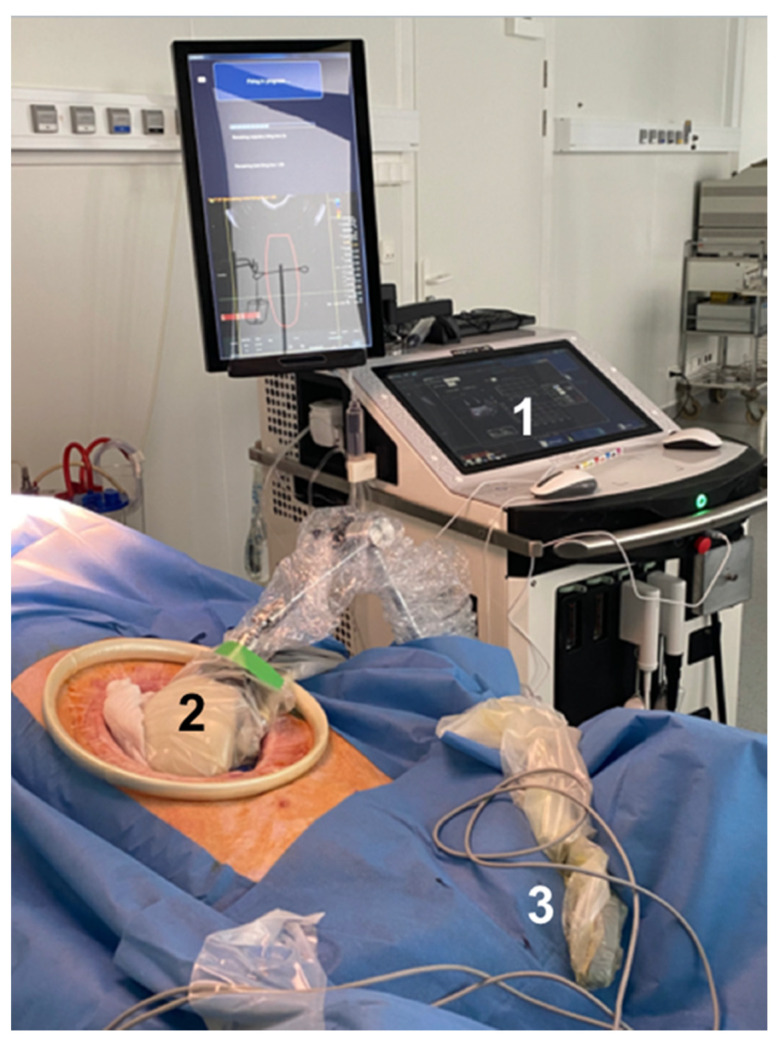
Experimental setup during in vivo treatment. 1: Electronic system used to control the HIFU transducer. 2: Intra-operative HIFU device. 3: Additional ultrasound imaging probe working at 14 MHz.
